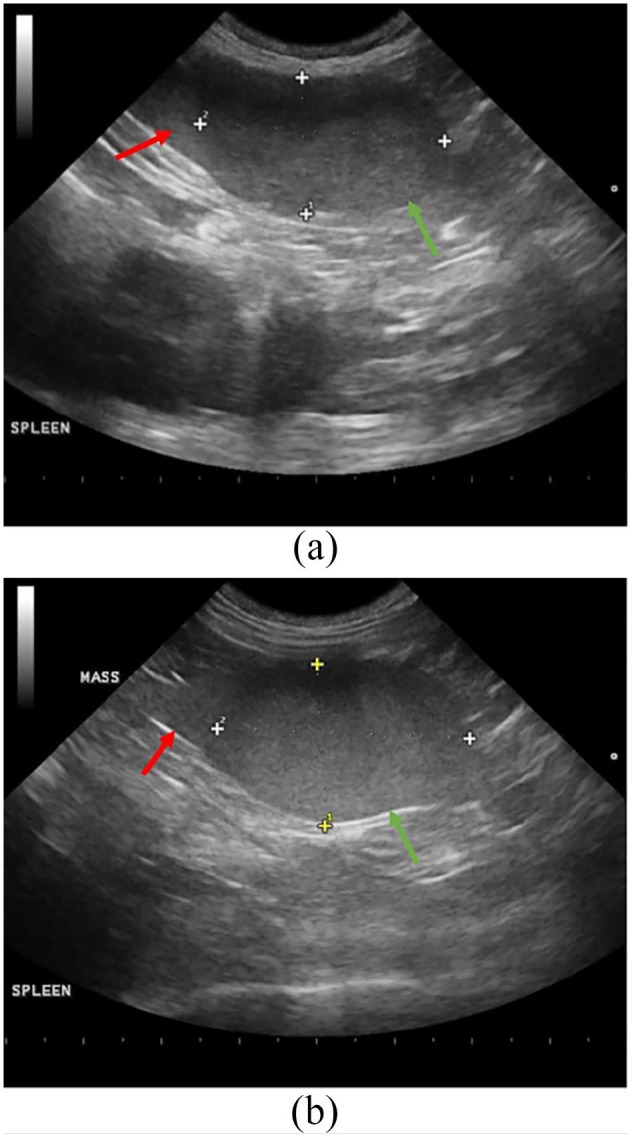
(a) Ultrasonographic image of the spleen on sagittal plane view at
